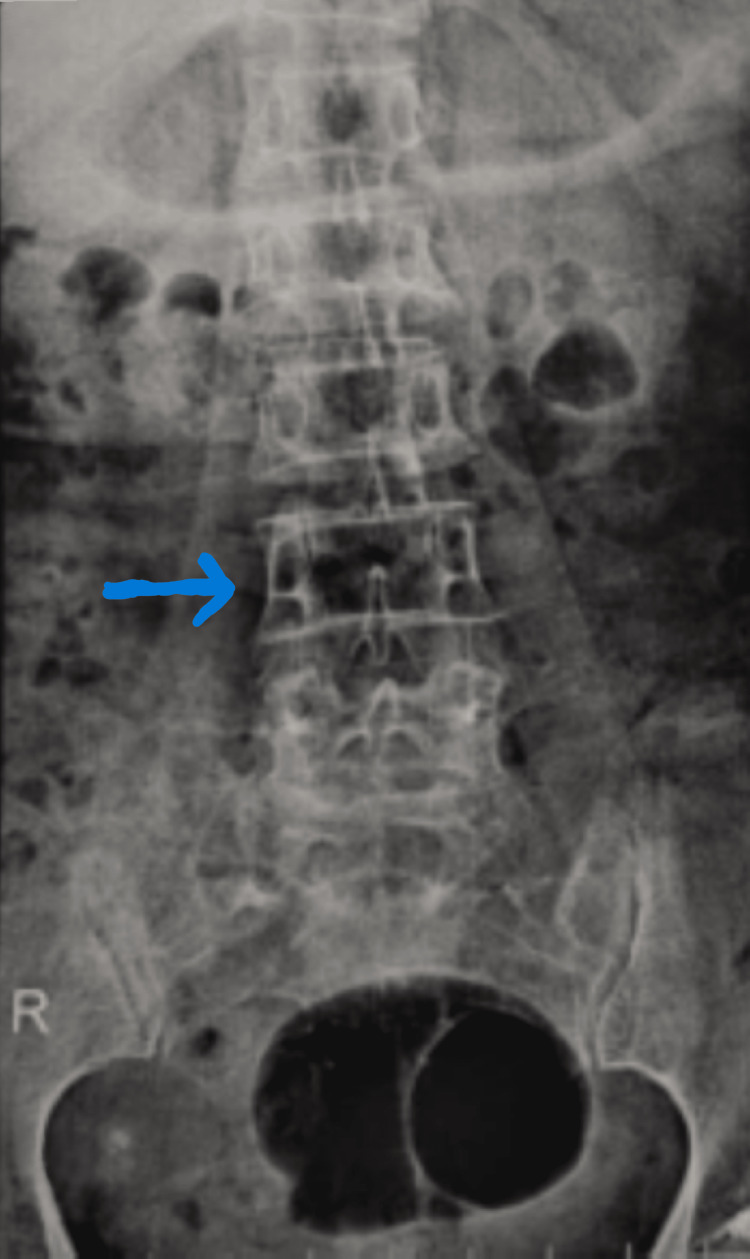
X-ray of the dorso-lumbar spine showing no signs of metastasis (anteroposterior view)
